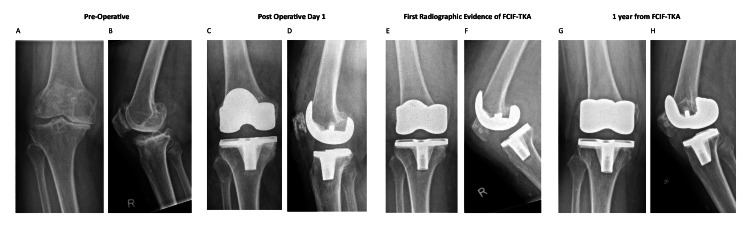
Patient 4 AP and lateral knee FCIF-TKA radiographs A: preoperative AP film, B: preoperative lateral film, C: postoperative day 1 AP film, D: postoperative day 1 lateral film, E: first radiographic evidence of FCIF-TKA AP film, F: first radiographic evidence of FCIF-TKA lateral film, G: one year from FCIF-TKA AP film, H: one year from FCIF-TKA lateral film
